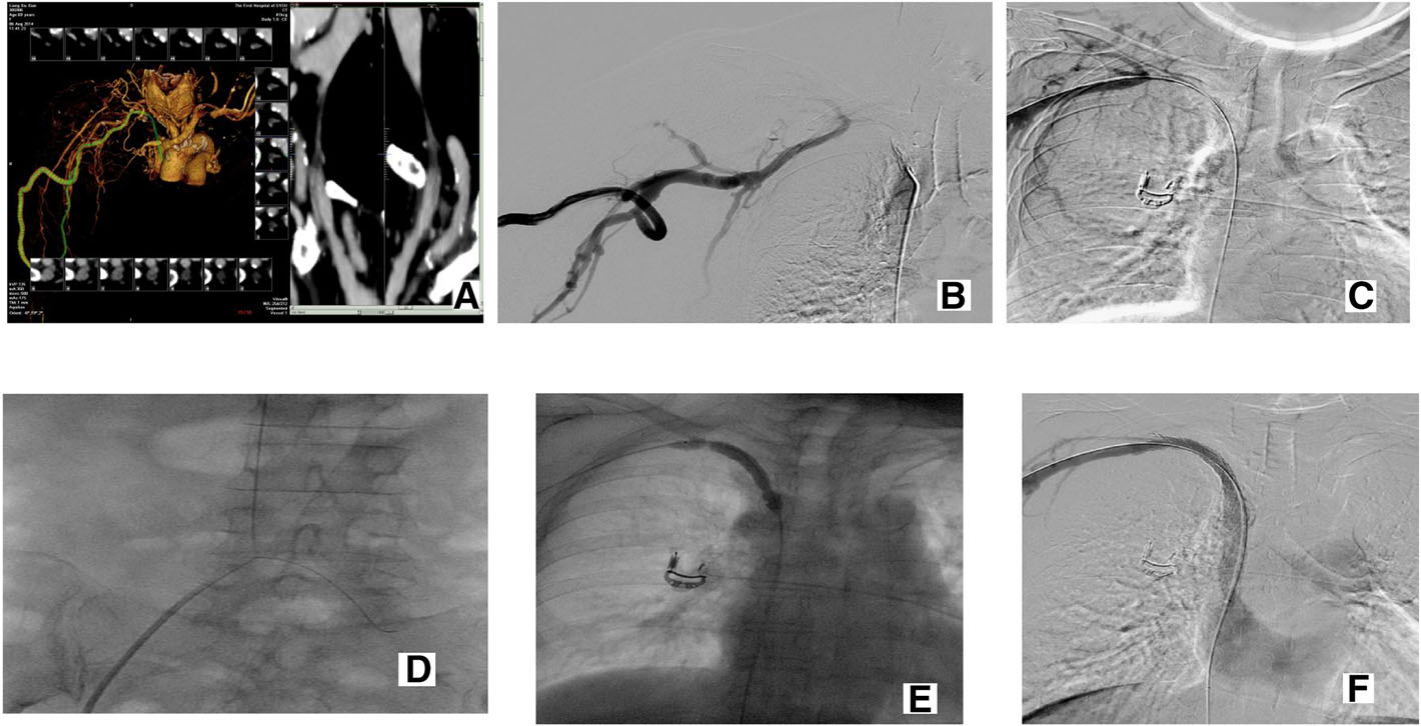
Dual venous access approach for a short occluded segment. The case had an occluded right innominate vein. a Preoperative evaluation of the length and extent of the occlusion by CT. b Synchronized angiography of the bilateral occluded ends further confirmed the length of occluded segment. c Guide wire was advanced via distal end of occluded segment. d Loop was pulled out from femoral vein sheath. e Balloon was dilated on the occluded segment. f Angiography showed successful recanalization after stent release
